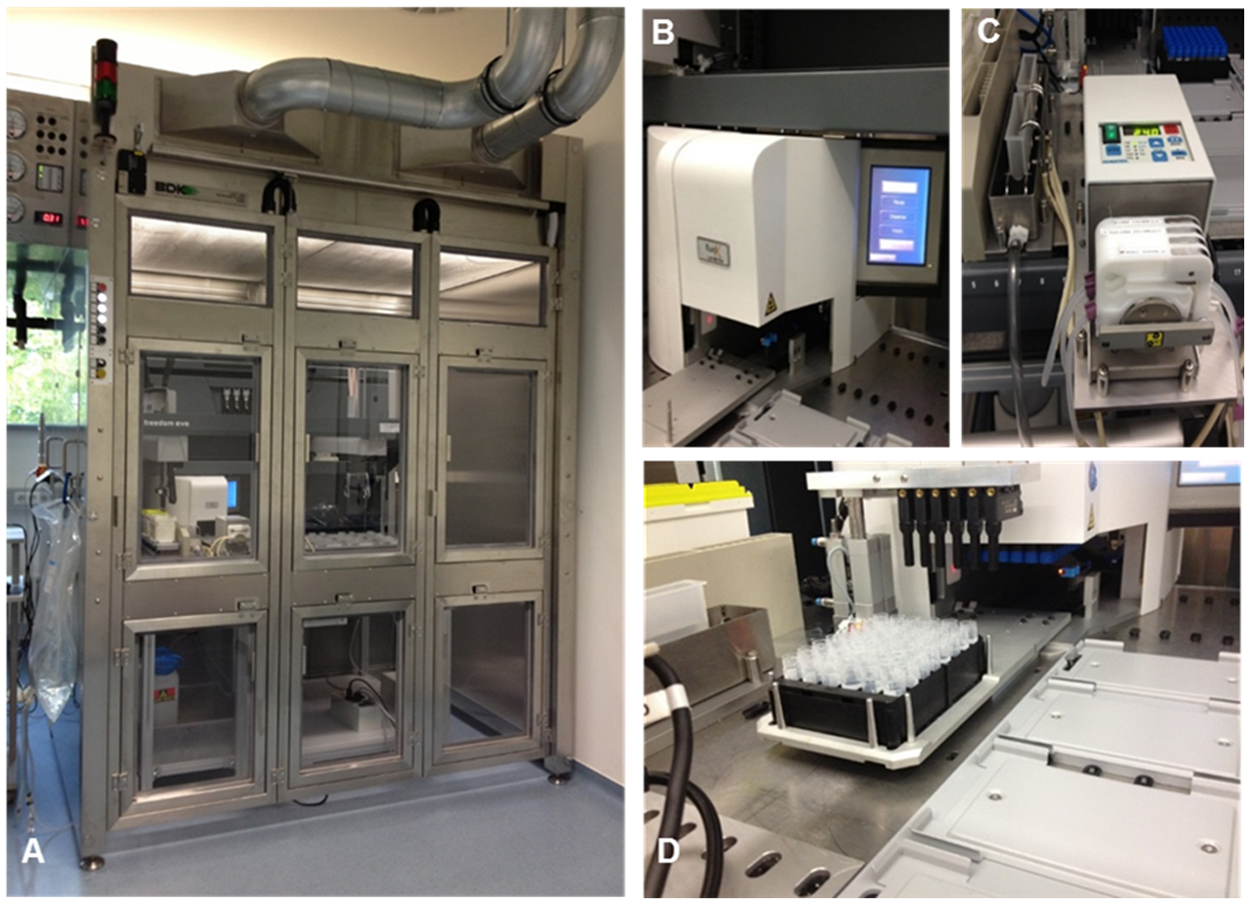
Automated system for aliquoting and the associated components. (A) Complete view of the system, (B) Decapper to open and close 48 cryovials simultaneously (C) Tubing pump mediating the transport of the virus containing solution from the virus supply bottle to the trough on the worktable, (D) at the back: ESD-system consisting of 6 ultrasound sensors integrated in the bridge for detecting the liquid level inside the tubes and the cap status; at the front: 48-way tube rack with 48 opened cryovials.
